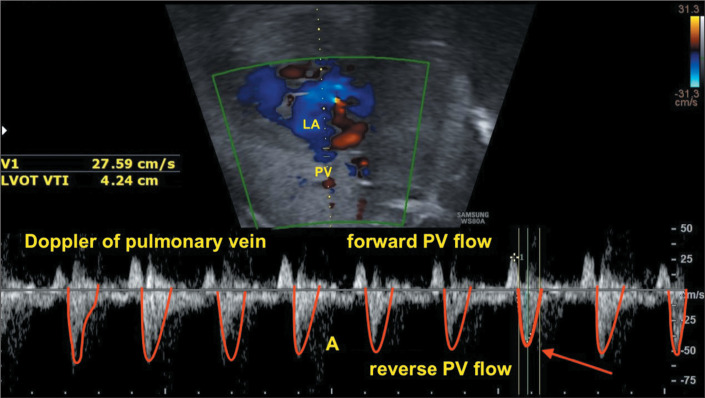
Doppler of the PV in a case of HLHS with restrictive FO. Doppler pattern
